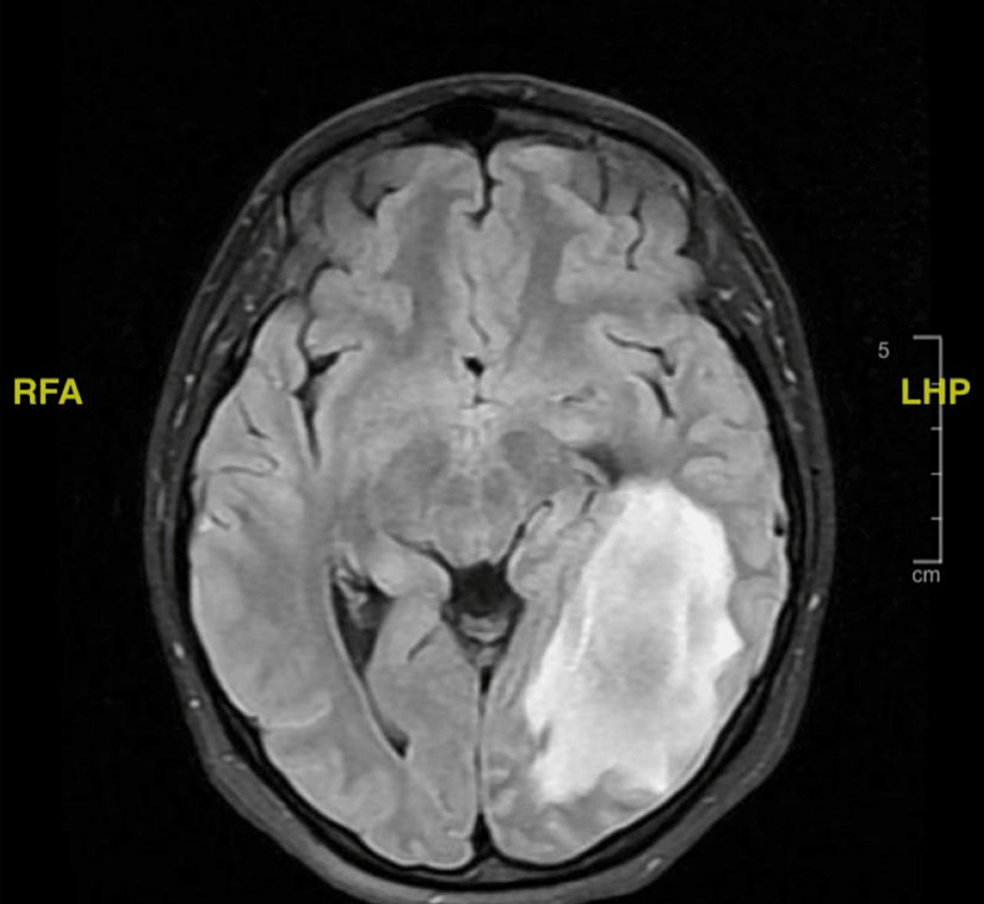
A FLAIR sequence MRI in axial view displaying a large left occipital space-occupying lesion with surrounding edema and peripheral diffusion restriction. FLAIR, fluid-attenuated inversion recovery; MRI, magnetic resonance imaging; LHP, left, head, posterior; RFA, right, foot, anterior
